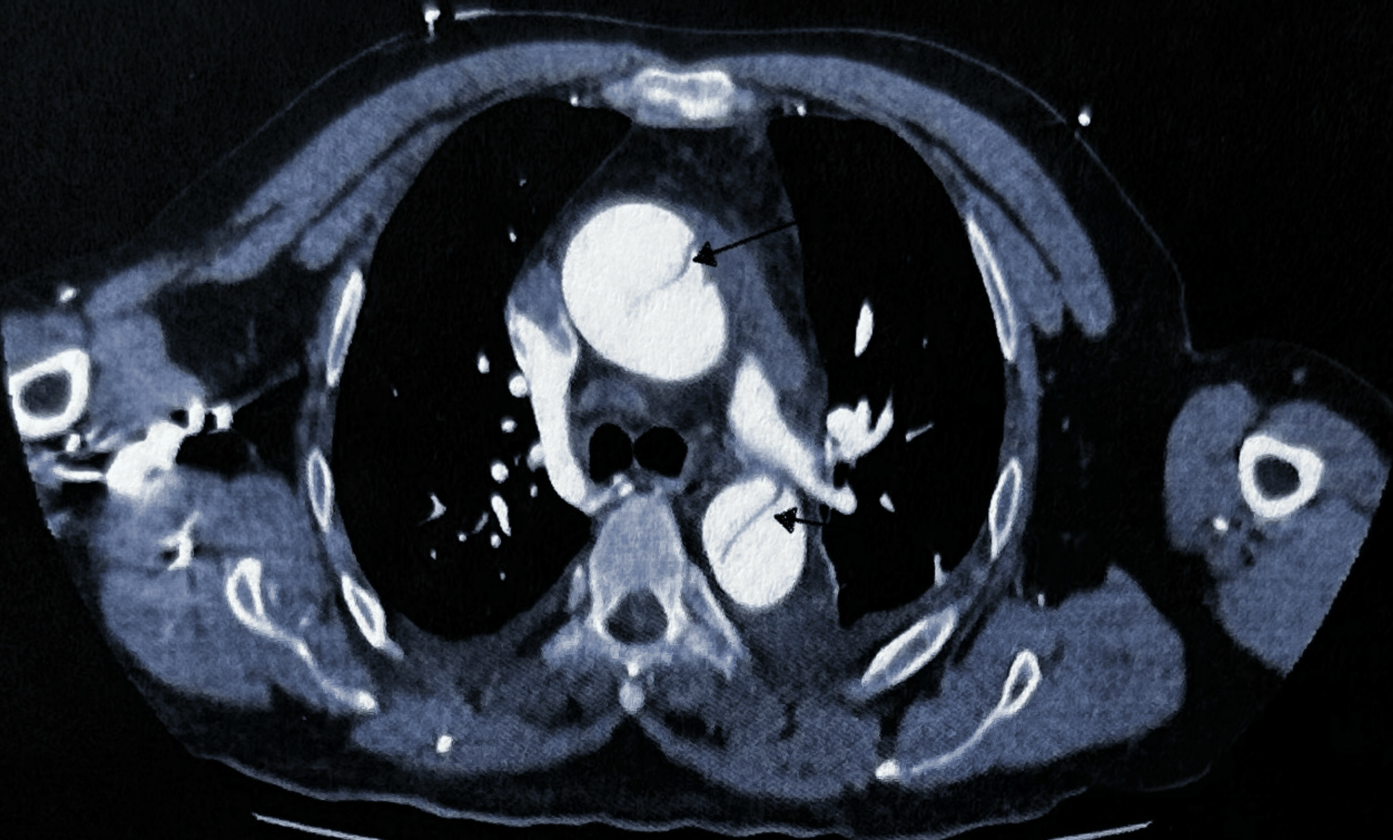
CT aortogram showing dissecting flaps in both the ascending and descending aorta.
